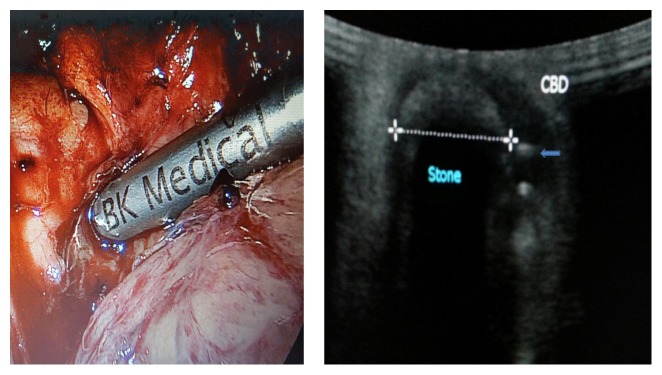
Laparoscopic ultrasonography probe utilized to determine location of remnant cystic duct stone and common bile duct stent (blue arrow).
